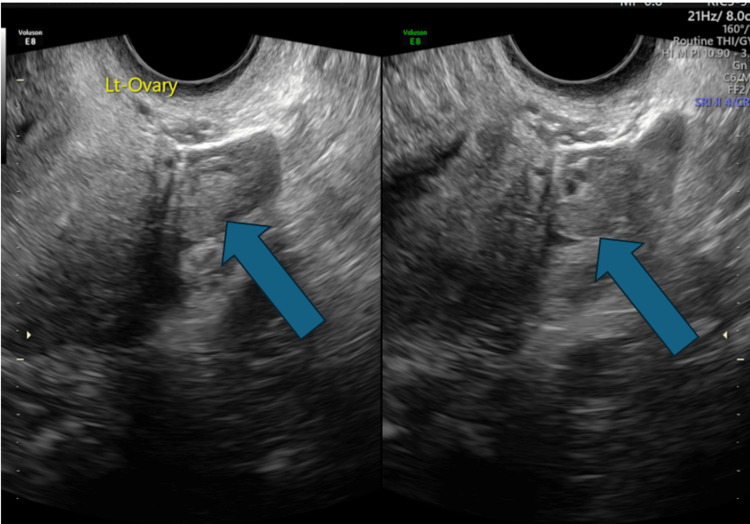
Transvaginal ultrasound: The left ovary (blue arrow) appears normal.
